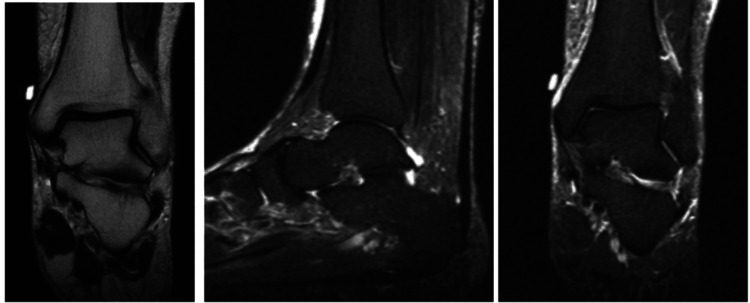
Initial MRI from February 8, 2022. No bone marrow edema or AVN and no fractures. AVN: avascular necrosis
